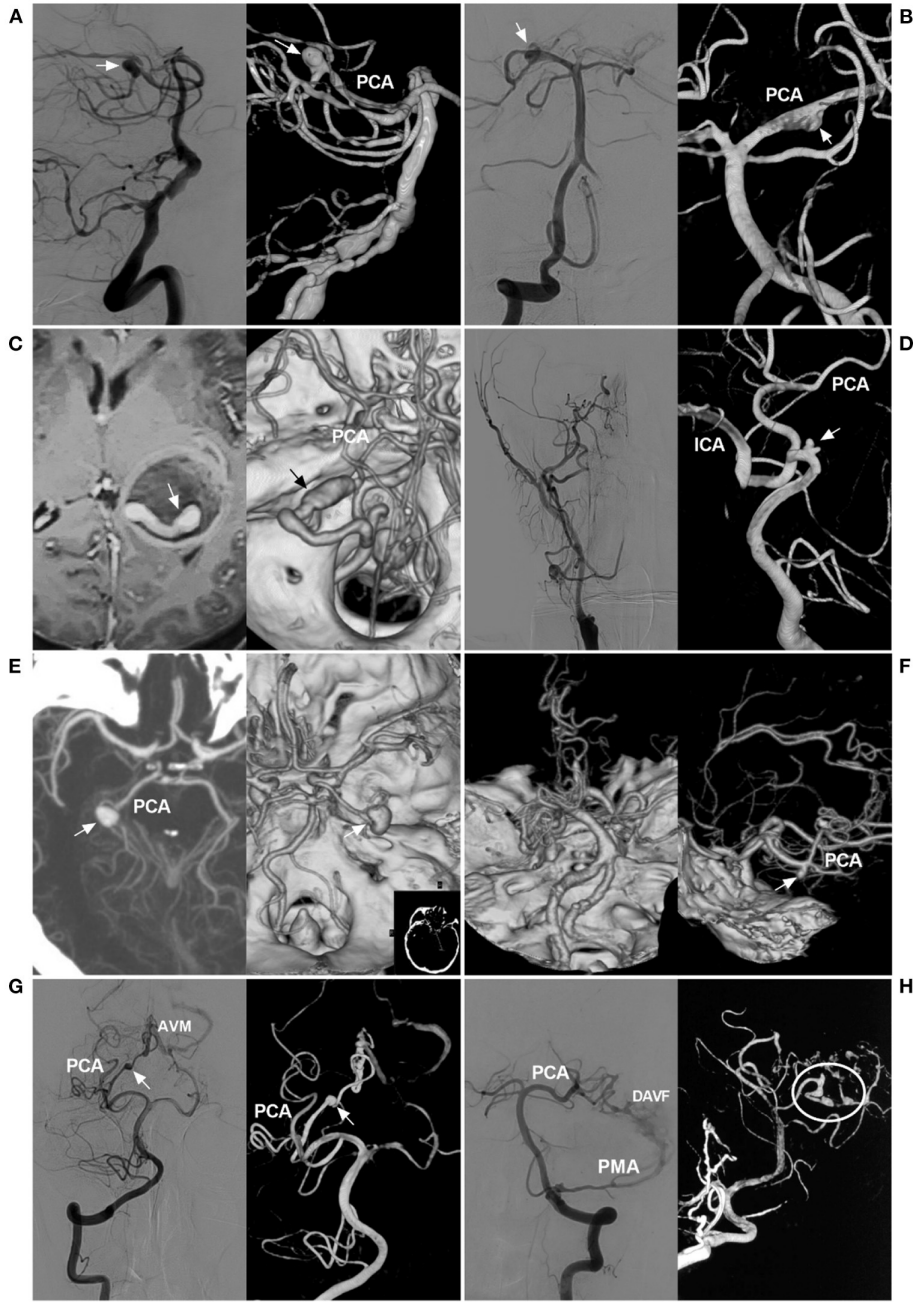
Classification of PCA trunk aneurysms. (A) Angiograms of the VA show a saccular true bifurcation aneurysm (arrows) at the distal segment of the PCA (left, two-dimensional angiogram; right, three-dimensional angiogram). (B) Angiograms of the VA show a dissecting aneurysm (arrows) at the proximal segment of the PCA (left, two-dimensional angiogram; right, three-dimensional angiogram). (C) MRI (left) shows an aneurysm with thrombosis [a tunnel can be seen (arrow)], and CTA (right) confirms a serpentine aneurysm (arrow) of the distal PCA. (D) Angiogram of the carotid artery (left) shows that the ICA was occluded at the beginning; three-dimensional angiogram of the VA (right) shows an aneurysm (arrow) located on the P1 segment, and the PCA supplies the anterior circulation. (E) CTA shows a P2 aneurysm (arrows) (left, maximum intensity projection; right, constructive image). (F) Left, CTA of the superior-inferior view shows occlusion of the bilateral middle cerebral arteries, confirming the moyamoya disease diagnosis; right, CTA of the lateral view shows an aneurysm (arrow) on the distal PCA. (G) Angiograms of the VA show the aneurysm (arrows) on the PCA as the feeding artery for an AVM (left, two-dimensional angiogram; right, three-dimensional angiogram). (H) Angiogram of the VA (left, two-dimensional DSA) shows that the PCA and PMA supply the DAVF; angiogram of the VA (right, three-dimensional angiogram) shows multiple tandem aneurysms (circle) on the PCA. AVM, arteriovenous malformation; CTA, computed tomography angiography; DAVF, dural arteriovenous fistula; ICA, internal carotid artery; MRI, magnetic resonance imaging; PCA, posterior cerebellar artery; PMA, posterior meningeal artery; VA, vertebral artery.
